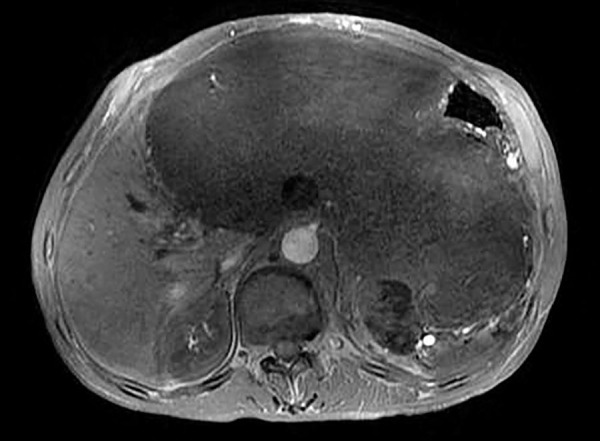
Inflammatory mass by MRI
